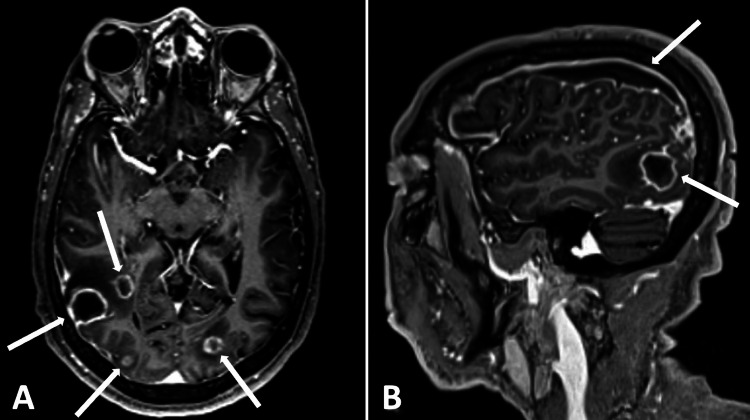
Initial brain MRI demonstrating multifocal ring-enhancing lesions in the parietal and occipital lobes, and right-sided subdural fluid collection. (A) Axial contrast-enhanced 3D MPRAGE; (B) sagittal contrast-enhanced 3D MPRAGE. 3D, three-dimensional; MPRAGE, magnetization-prepared rapid acquisition gradient echo.
